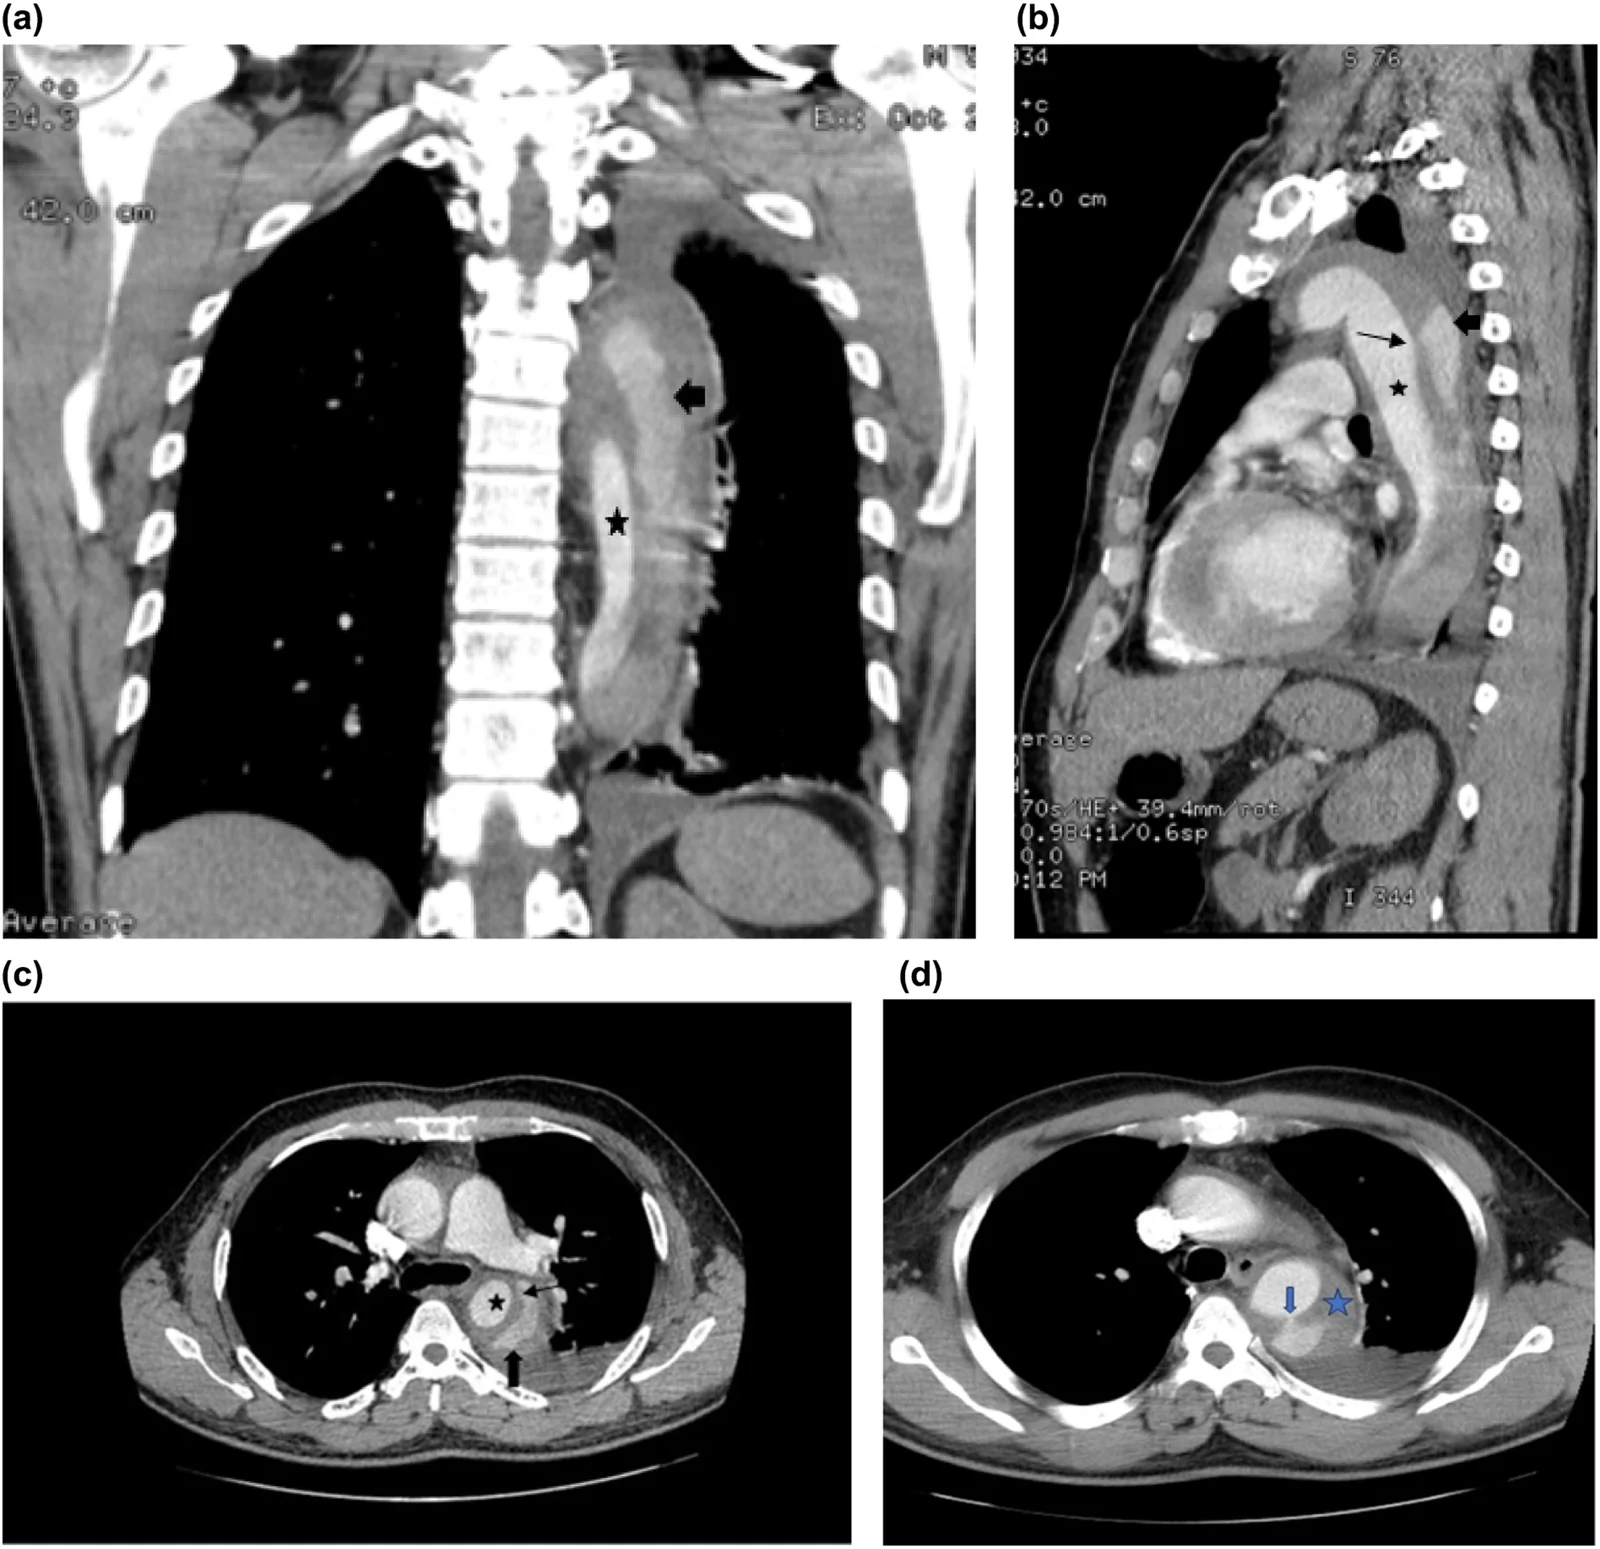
a: Post-contrast high-resolution coronal plane of chest CT scan revealing aortic dissection extending from the arch (after the left subclavian artery) to the descending thoracic aorta showing the true lumen (star) and false lumen (big arrow) and the presence of adjoining mural thrombi. b: Post-contrast sagittal chest CT scan of the aortic arch and descending thoracic aorta revealing an intimal flap (small arrow) separating the true (star) and false lumens (big arrow) and the presence of adjoining mural thrombi. c: Post-contrast axial chest CT scan of the descending thoracic aorta revealing an intimal flap (small arrow) separating the true (star) and false lumens (big arrow), and the presence of adjoining mural thrombi. d: Post-contrast axial chest CT of the descending thoracic aorta highlighting the communication ‘entry tear’ (arrow) between the true and false lumens and the presence of adjoining mural thrombi (star)
